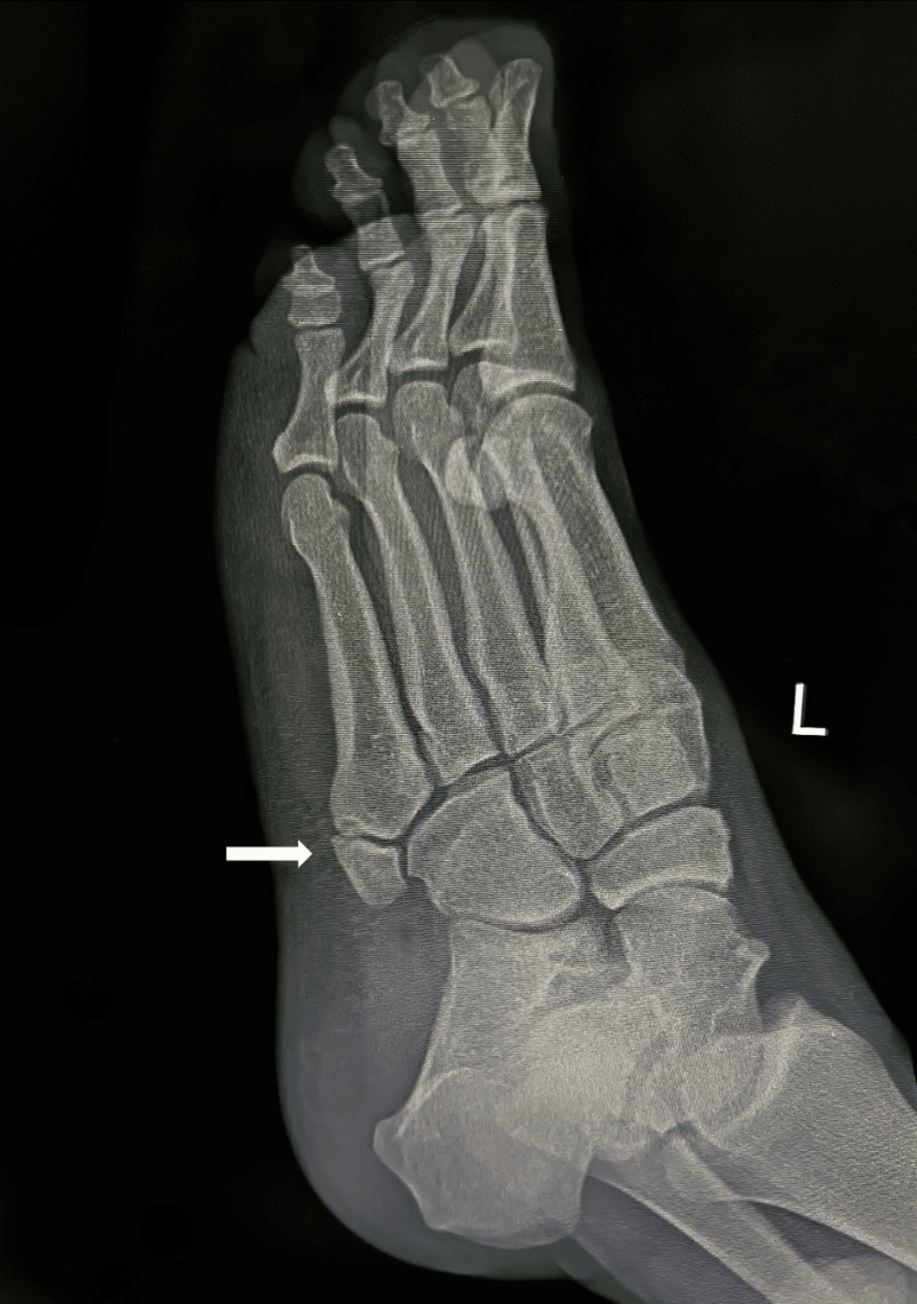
Ankle X-ray revealing the presence of an OVP bilaterally (left) OVP, os vesalianum pedis
